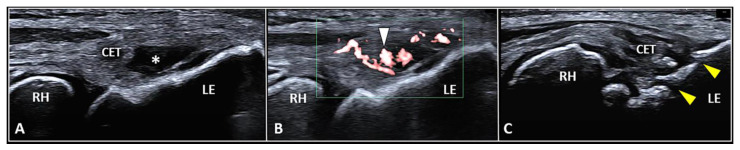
Partial tear (*white asterisk*) of the insertion zone of CET (A), focal tendinosis with inner hypervascularization (*white arrowhead*) (B), and enthesitis with cortical irregularities (*yellow arrowhead*) (C) of the lateral epicondyle (*LE*) should be considered among the most common sonographic findings related to the clinical picture of lateral epicondylitis. RH: radial head.
